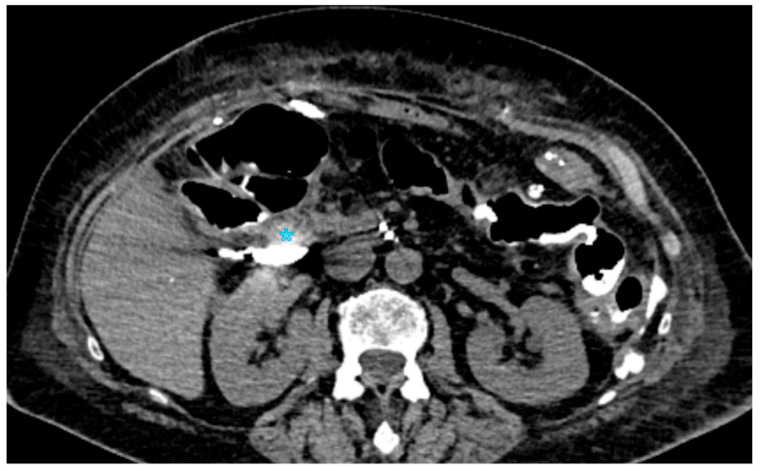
CT cholangiography through a Kehr tube showing the spread of contrast medium (blue asterisk) in the subhepatic region, anterior to the kidney, compatible with a biliary leak in a patient who underwent duodenopancreatectomy 5 days earlier.
